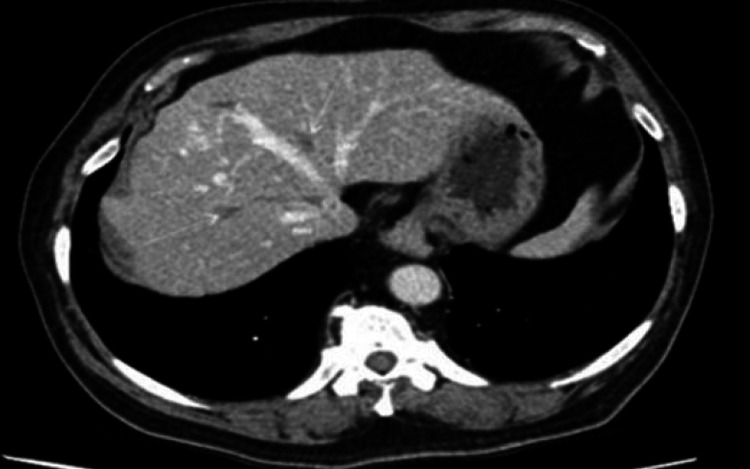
CT abdomen cross-sectional view The image shows complete resolution of stomach cancer with complete disappearance of metastasis along the liver and portal area six years after the initial presentation CT: computed tomography
